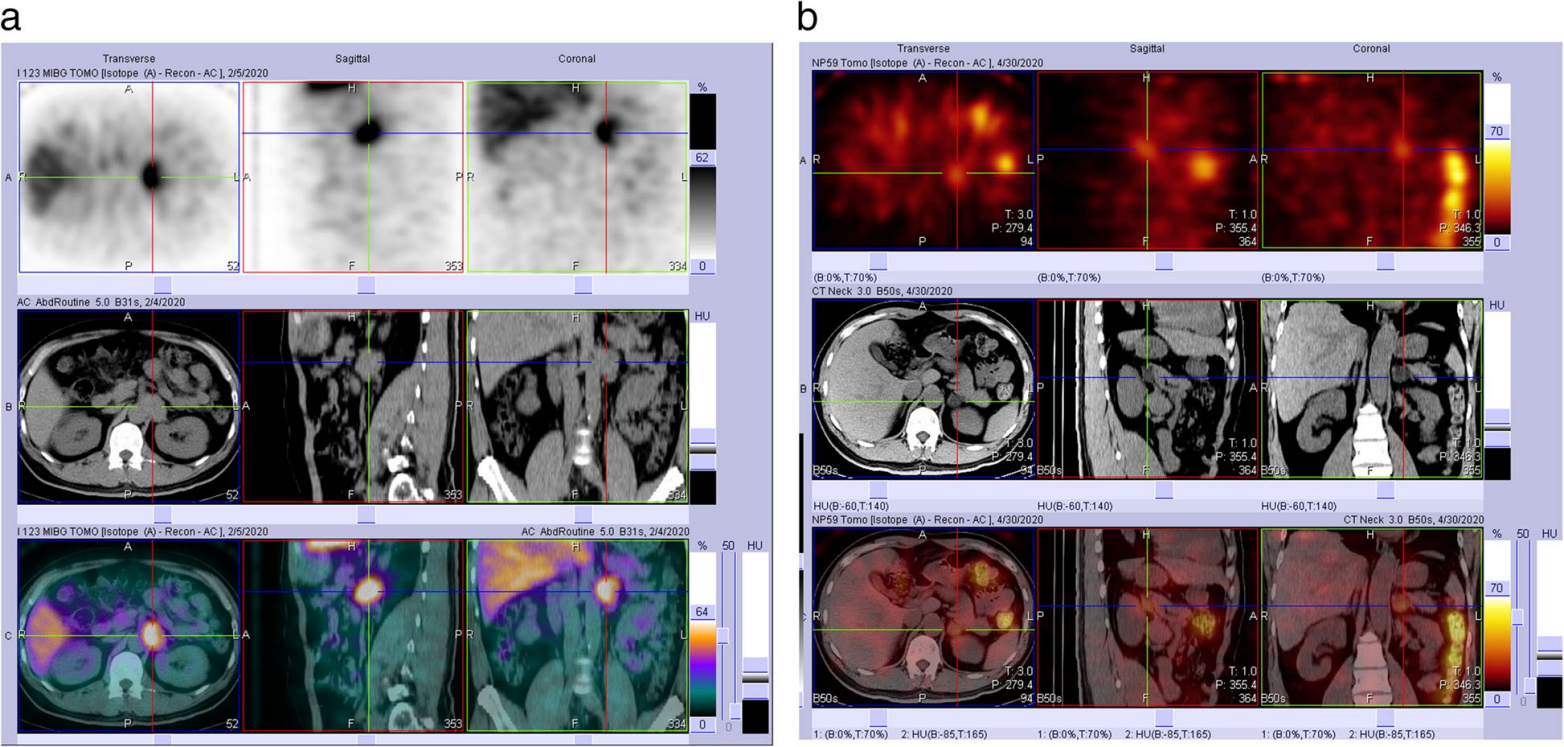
Metaiodobenzylguanidine scan (a) showed uptake over the left para-aortic tumor, which favored paraganglioma, and NP-59 adrenal scintigraphy (b) showed mild radiotracer accumulation over the left supra-renal region, which favored left adrenal functional cortical tumor
